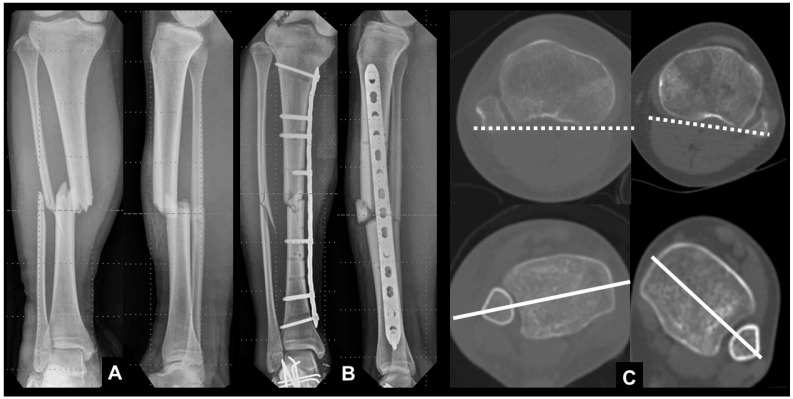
(A) A 15-year-old girl with a tibia shaft fracture; (B) minimally invasive plate osteosynthesis was carried out; (C) postoperative CT scan showing a moderate difference (over 16 degrees) in rotation compared with the noninjured side.
